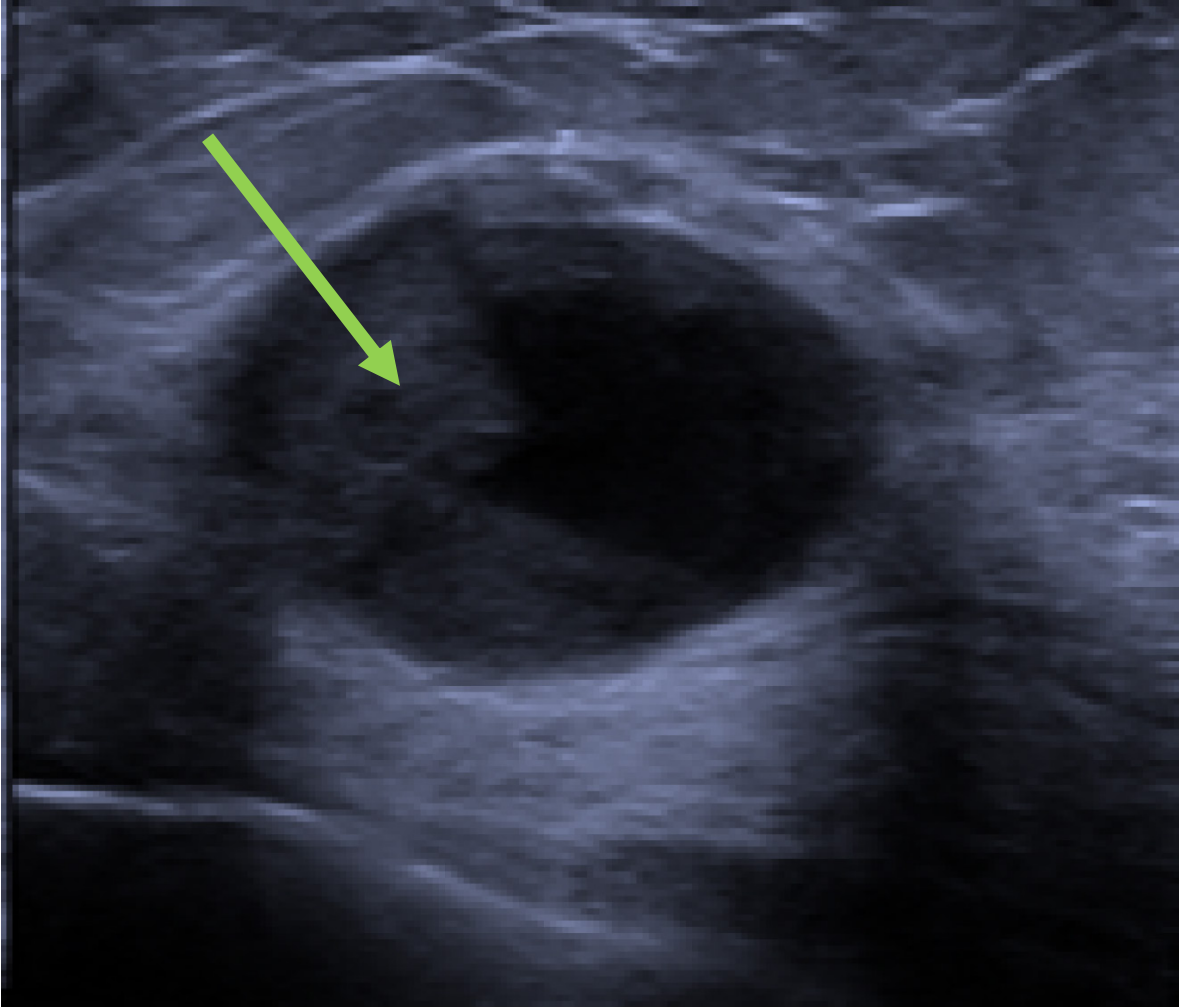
Ultrasound image showing a complex solid‐cystic mass containing an eccentric mural solid component. The margins are partly ill‐defined.
